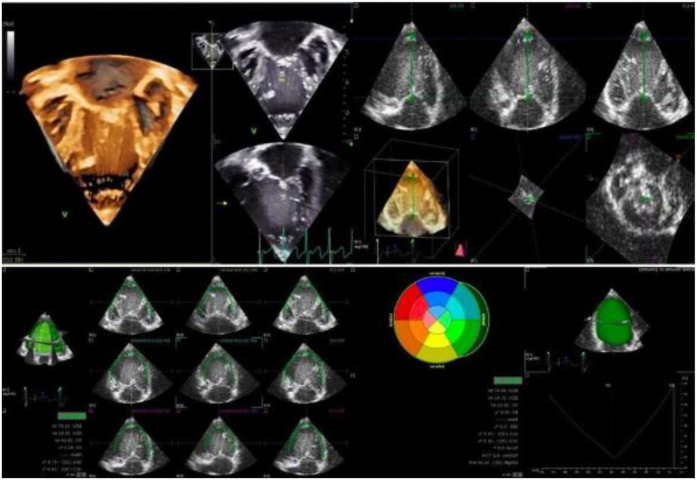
A full 3-dimensional volume is captured from a apical four-chamber view. The atrioventricular valve, apex and aorta/neoaorta is identified and the endocardial border is traced to calculate volumes, ejection fraction and strains.
